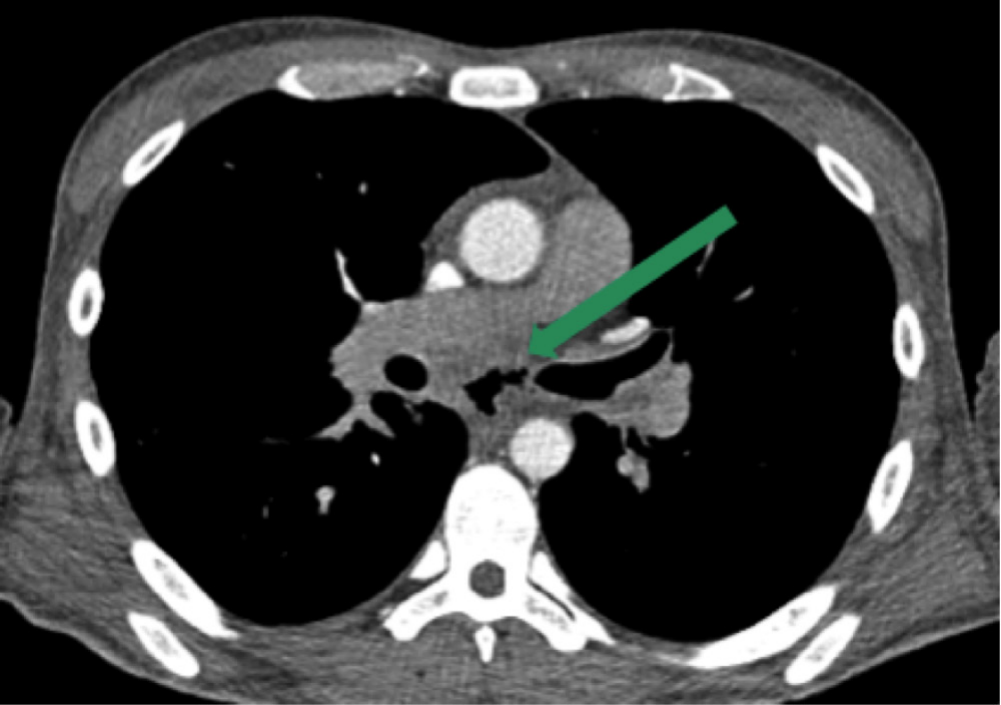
Chest CT on admission revealed esophageal discontinuity in the subcarinal region representing esophageal perforation (arrow).
